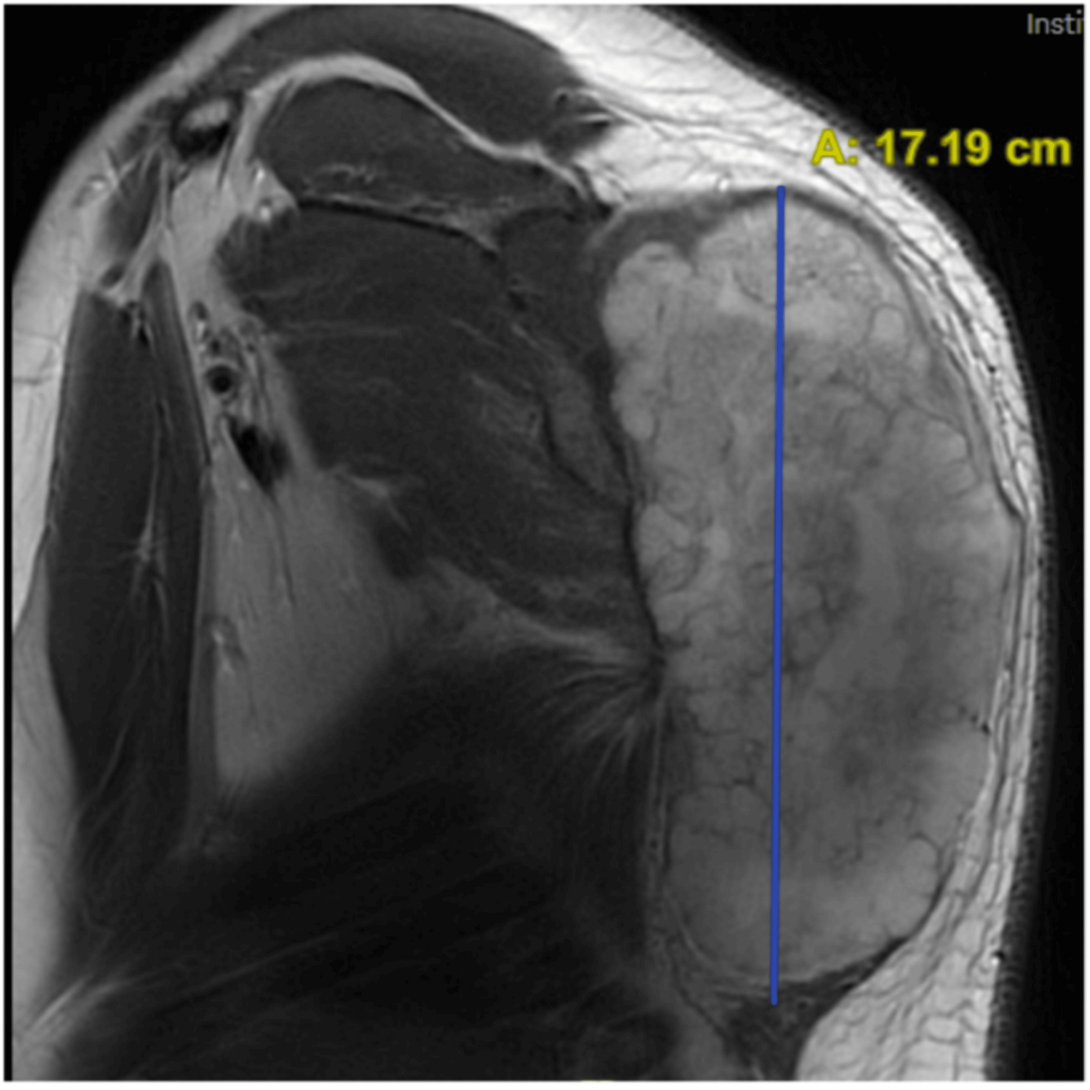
Preoperative sagittal MRI view. The tumor measured 17.91 cm in the maximum superior to the inferior axis.
